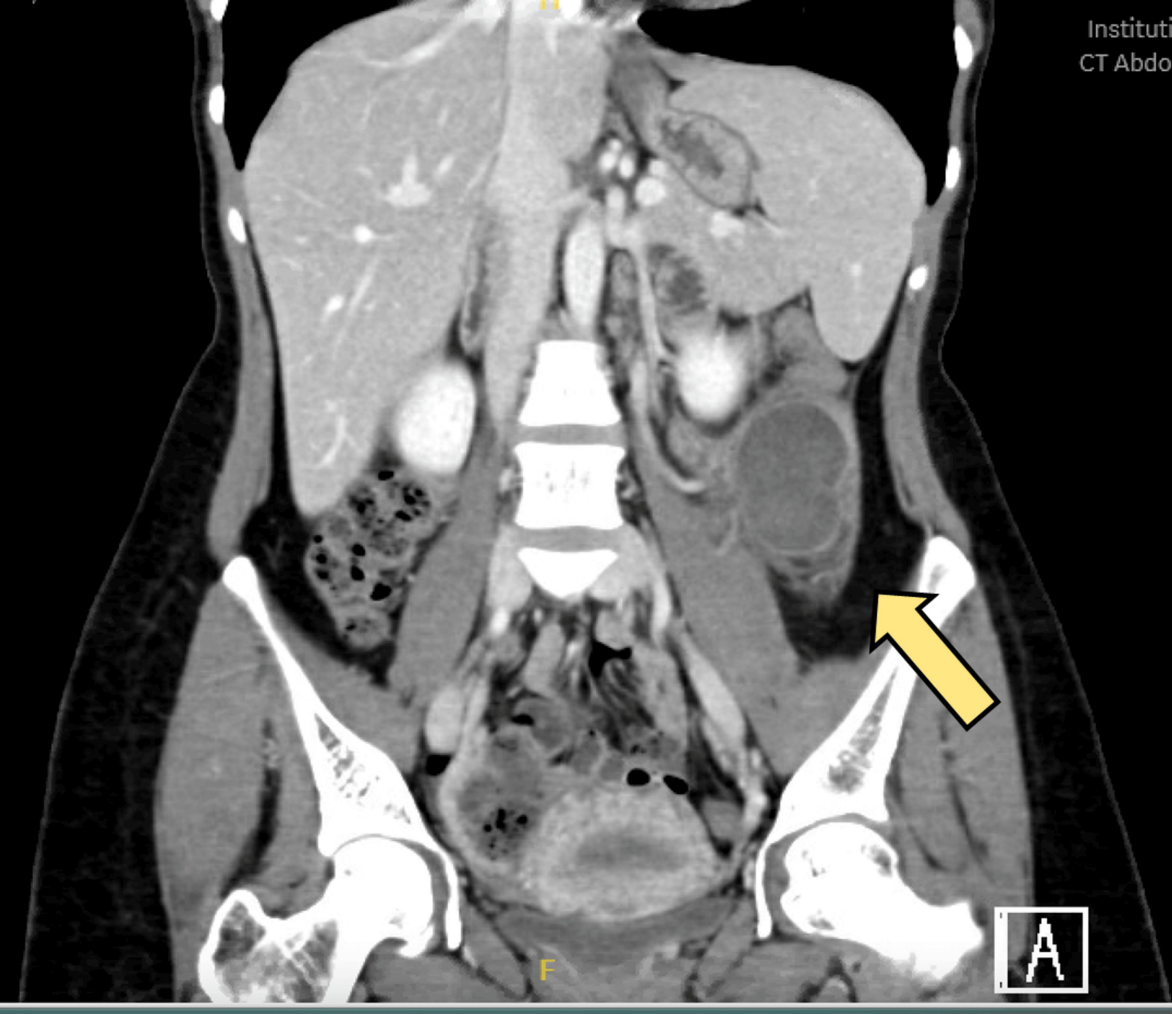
Coronal view CT scan showing left sided colonic mass The yellow arrow indicates the left-sided colonic mass.
